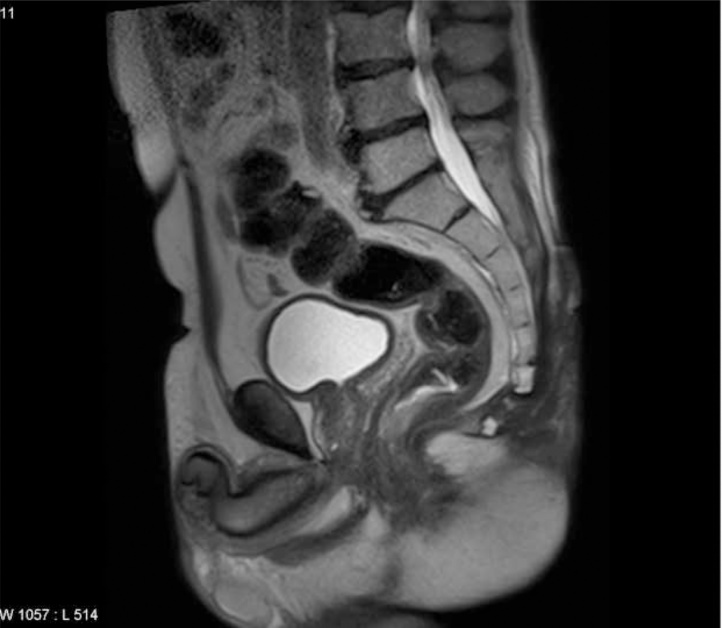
MRI imaging after neoadjuvant treatment(sagital view).
